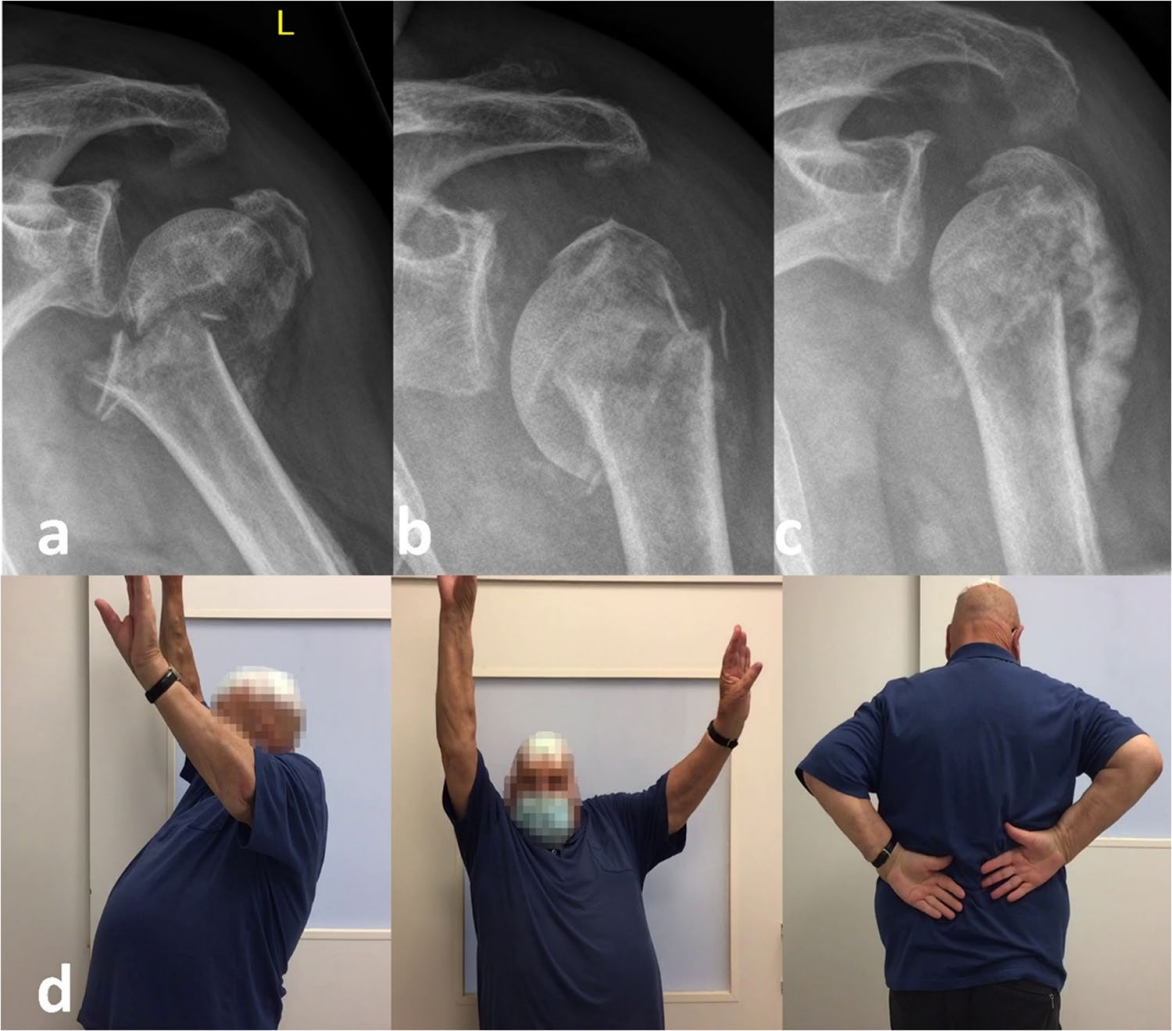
AP radiographs of a 79-year-old patient with considerable medial displacement of humeral shaft with metaphyseal comminution and significant valgus displacement of the humeral head (a). Without any reduction maneuvers or medical aids, orthograde self-reducing of the humeral shaft was observed after 6 weeks, however also secondary varus displacement of the humeral head (b). Despite this, nonperative treatment was continued with decreasing pain level. After 6 months fracture healing in nonanatomic position (c) with nevertheless good functional outcome (subjective shoulder score: 80%, absolute Constant Score: 75 out of 100 points, no pain; d, e, f). Reprinted with changes from *“Algorithmus zur konservativen Behandlung von proximalen Humerusfrakturen”* (https://link.springer.com/article/10.1007/s11678-022-00702-y, Copyright© 2022, Razaeian) under the terms of the Creative Commons CC BY 4.0 license (http://creativecommons.org/licenses/by/4.0/deed.de)
